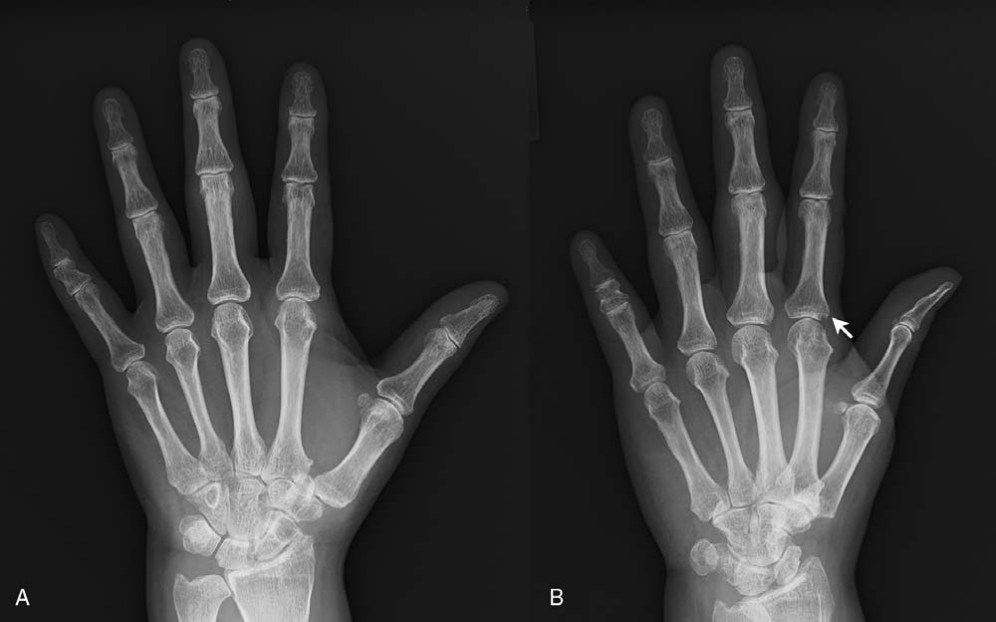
X-rays of the left hand (A: posteroanterior view and B: oblique view) a year after the diagnosis of RA. An erosion is seen in the 2^nd^ metacarpophalangeal joint, indicated with a white arrow. RA = rheumatoid arthritis.
